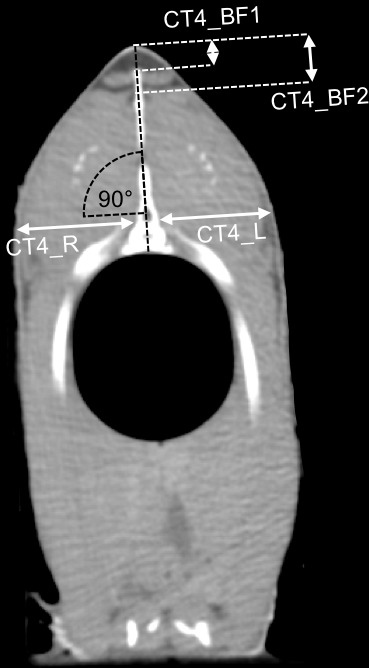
CT cross section 2.1 cm before the first ray of the dorsal fin.
